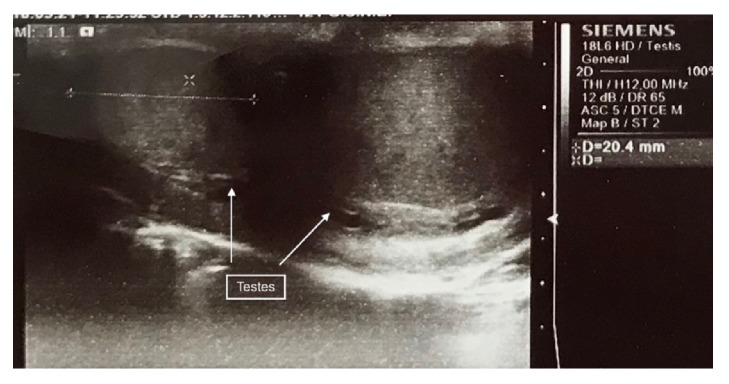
Sagittal ultrasound image depicting two testes in the same hemiscrotum.
